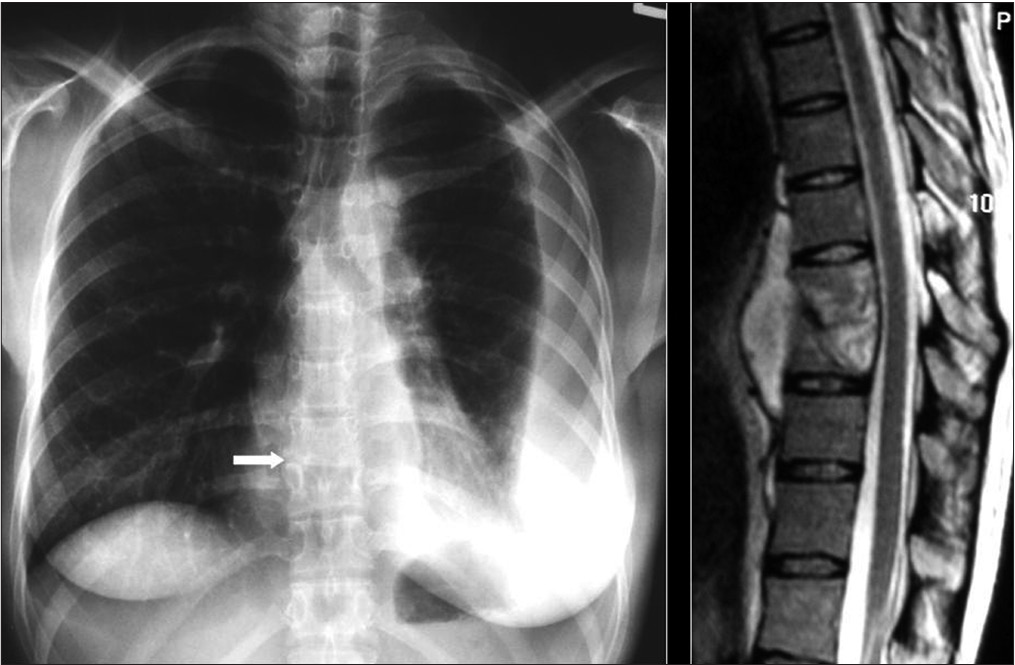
A 26-year-old human immunodeficiency virus-positive female presented with shortness of breath. The chest X-ray shows a large left-sided pleural effusion and loss of height and erosion of the articular plates between the 9^th^ and 10^th^ vertebral bodies associated with soft tissue swelling. A sagittal T2-weigted magnetic resonance scan of the dorsal spine shows complete obliteration of the disc between the 9^th^ and 10^th^ dorsal vertebral bodies associated with fluid collection anterior to the spine representing pus. Acid-fast bacilli were identified in the aspirated pus
